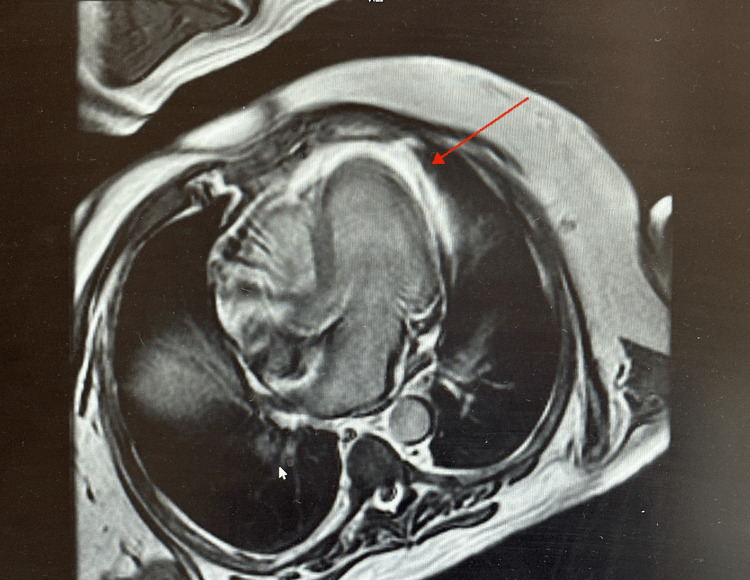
Cardiac MRI (T2 weighted STIR) showing increased signal intensities in the pericardium due to pericardial effusion (red arrow) which in the context of myocarditis suggests pericardial inflammation STIR: Short tau inversion recovery
